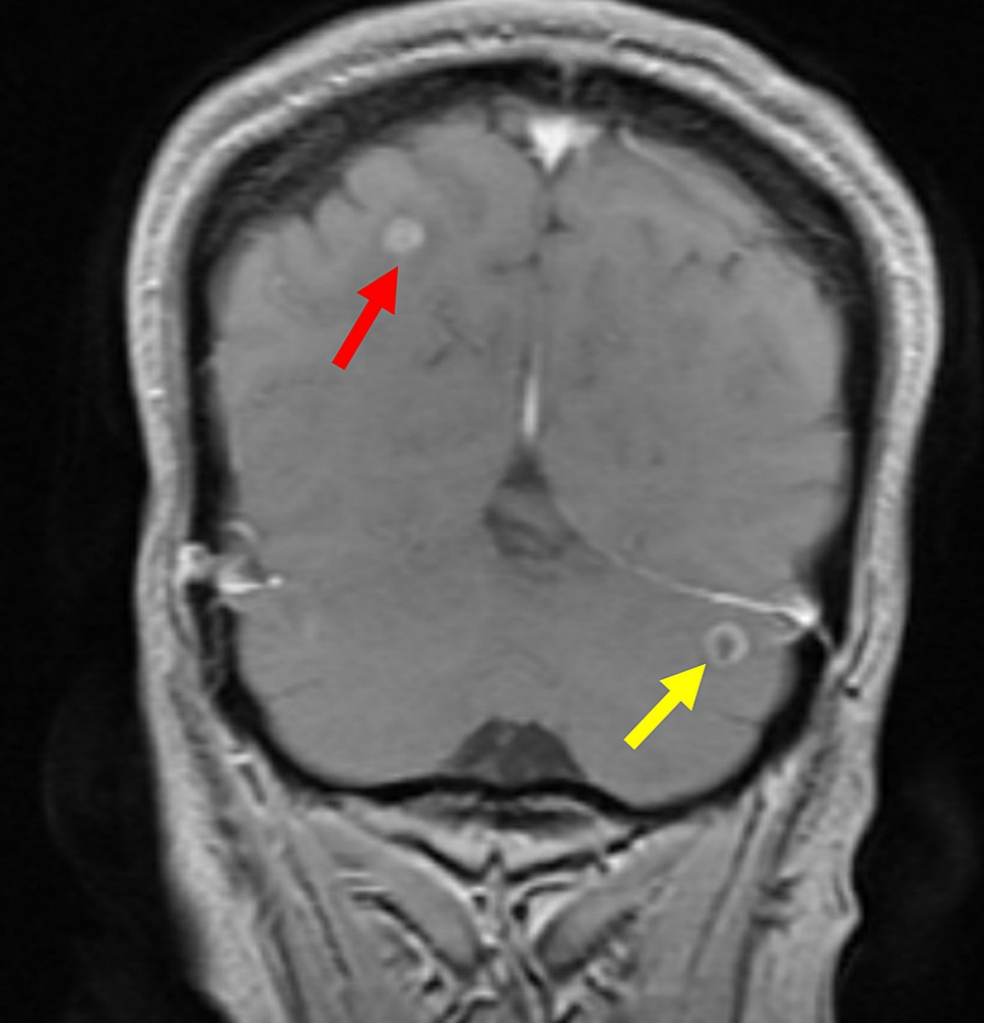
MRI showing metastatic lesions in the cerebrum (red arrow) and cerebellum (yellow arrow).
